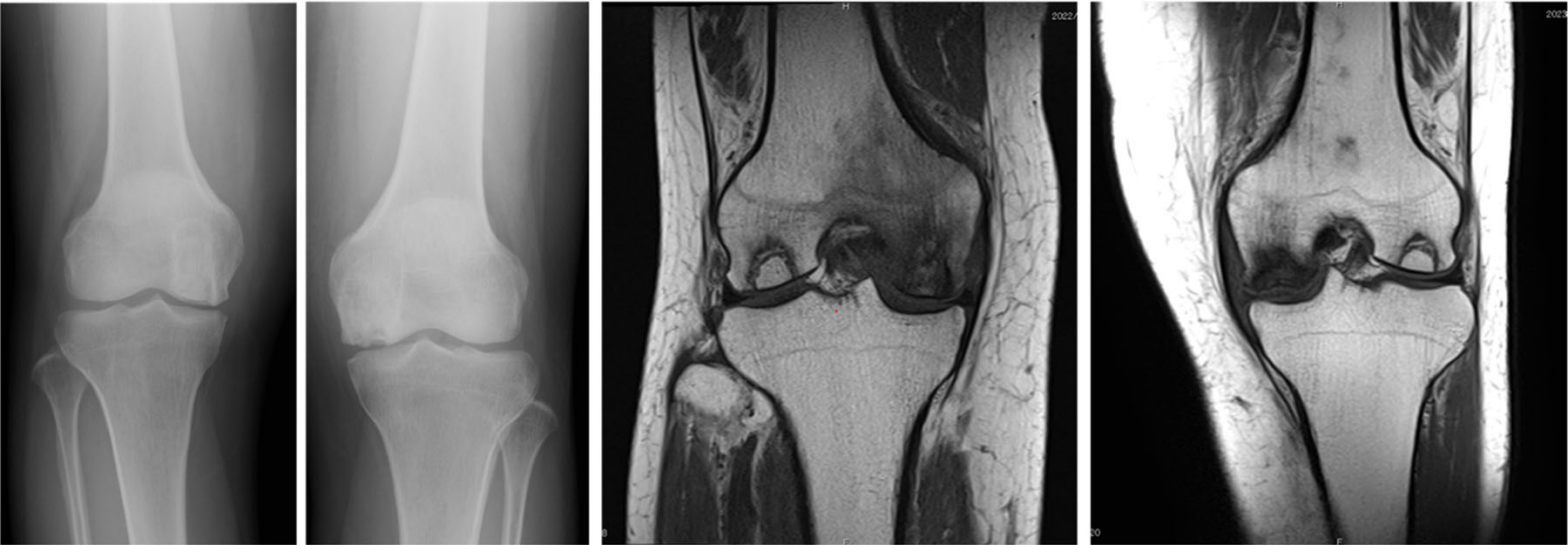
Plain radiographs and magnetic resonance imaging of corticosteroid‐associated bilateral knee osteonecrosis in a 42‐year‐old female patient.
